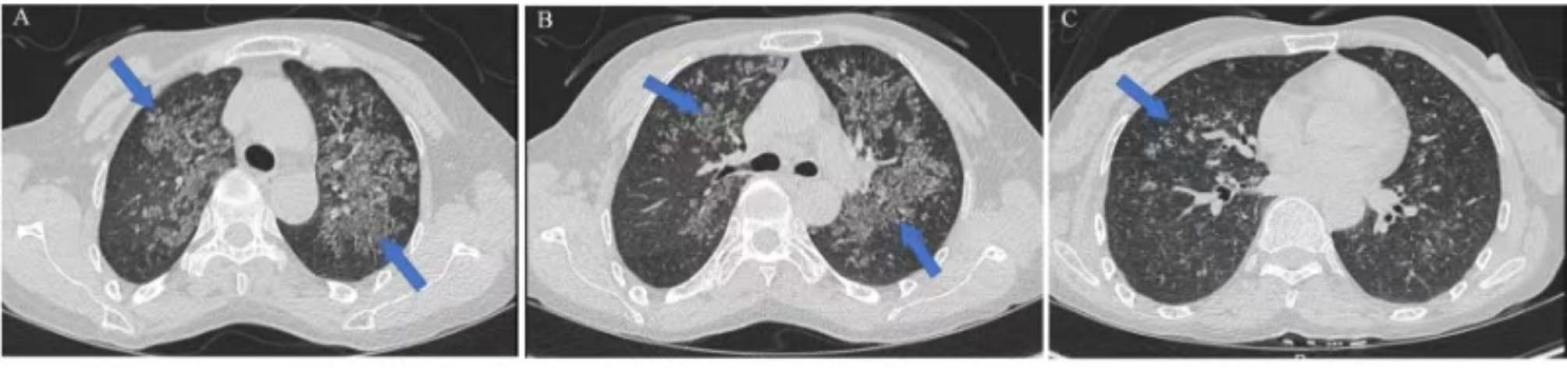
Chest CT scan of patient 1. A-C. The different layers of chest CT images at the time of patient 1 show diffusely distributed multiple patchy shadows in both lungs with unclear boundaries (blue arrow)
